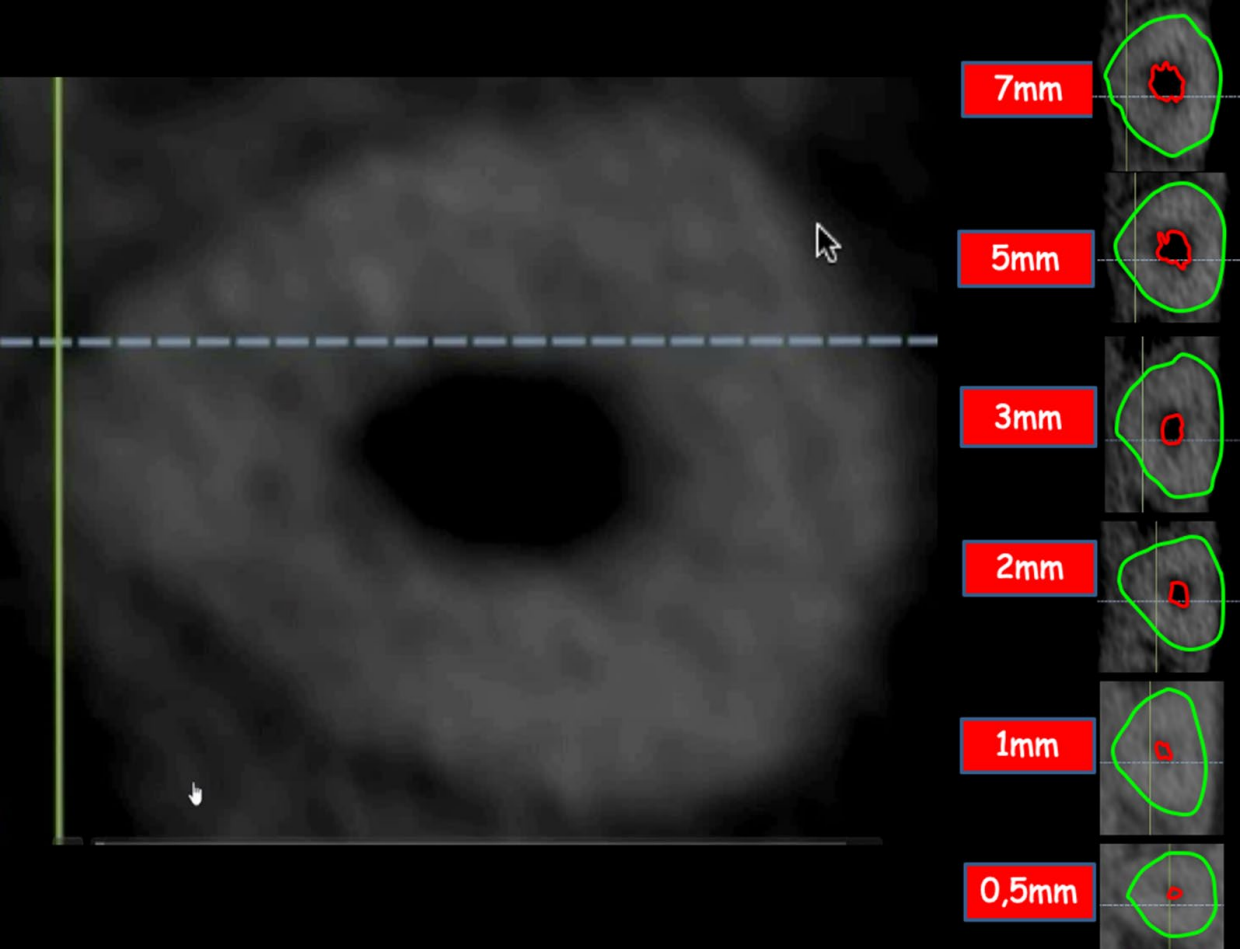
CBCT axial plane slices made at 0.5 mm, 1 mm, 2 mm, 3 mm, 4 mm, 5 mm, 6 mm and 7 mm, where measures of the root canal and the root were taken for three-dimensional reconstruction. Snapshot images of the different sections were imported with the RHINOCEROS 5.0 3D software to draw the canal and the root
